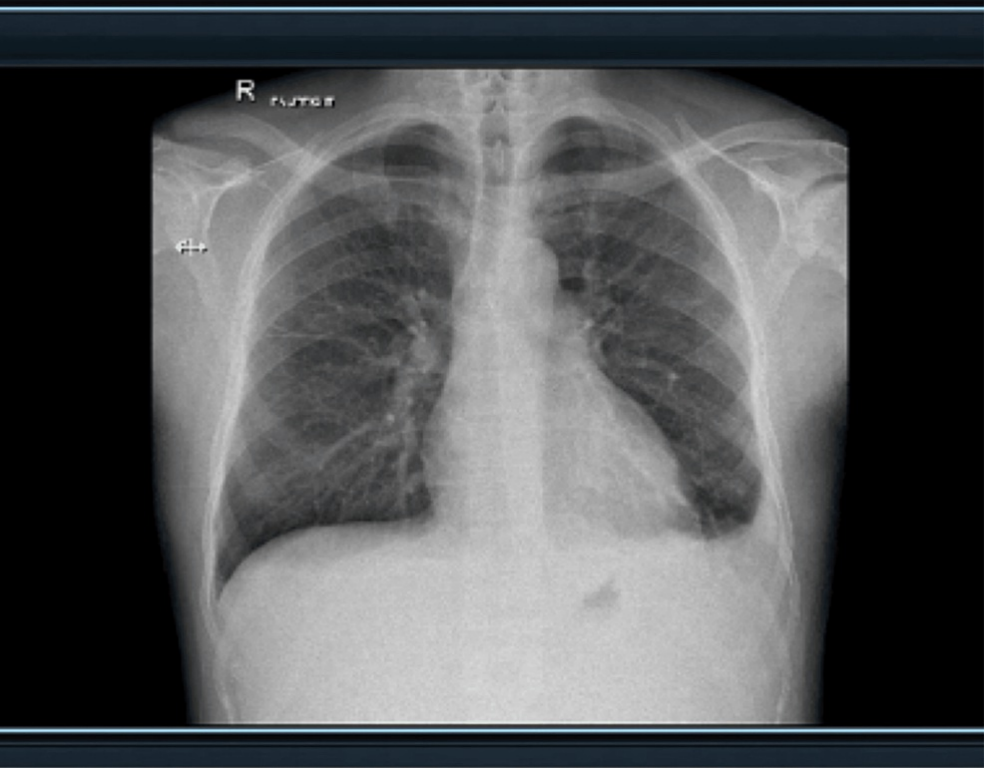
Chest X-ray showing clear lungs with no active or significant pathology. No hyperinflation or pneumothorax and no free subphrenic gas
